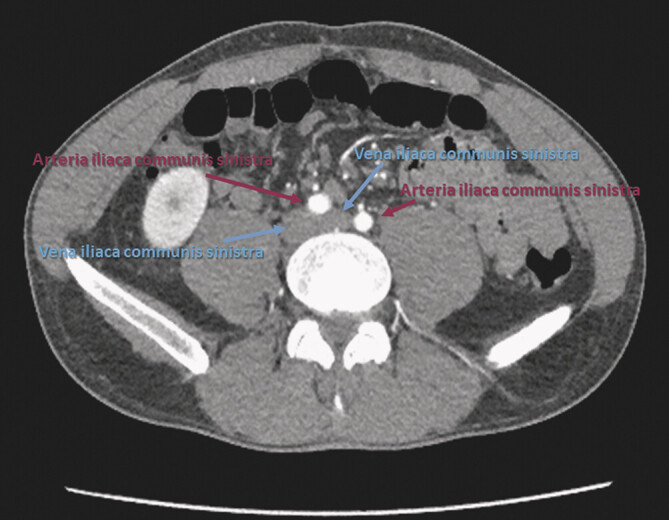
CT des Abdomens – Transversalscan (axiale Rekonstruktion mit arterieller Kontrastmittelphase): May-Turner-Syndrom infolge der Kompression der Beckenvenen durch die Beckenarterien auf dem Lendenwirbelkörper mit Ausbildung eines Beckenvenensporns.
